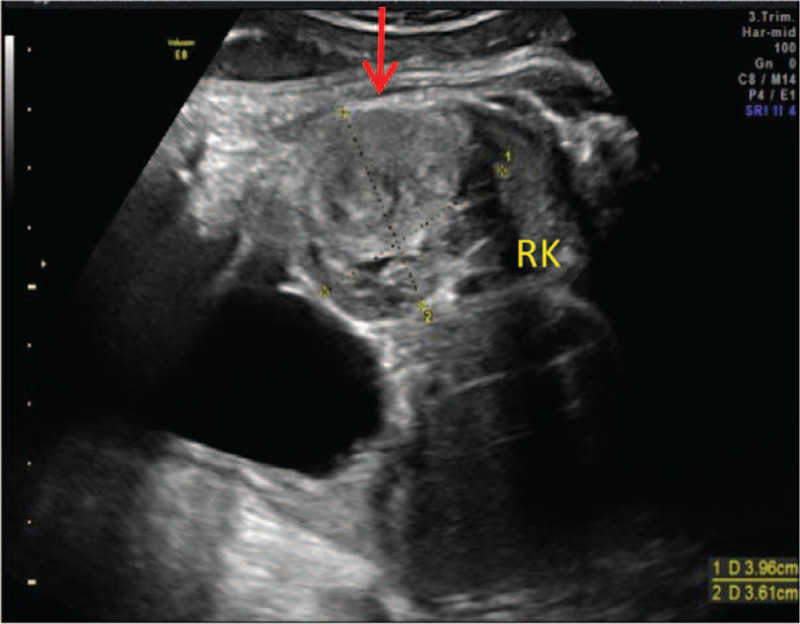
Prenatal ultrasonography detection indicates a 3 × 3 cm solid, well-defined, hyperechoic mass with uniform internal echo in the fetal right kidney.
